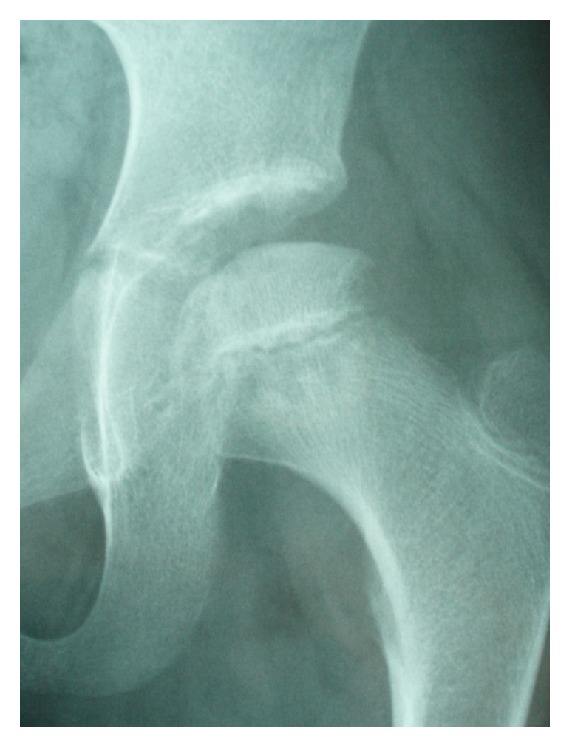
Plain radiograph of the hip joint 3 months after the initial visit. Bone resorption was observed at the left femoral capital epiphysis.
